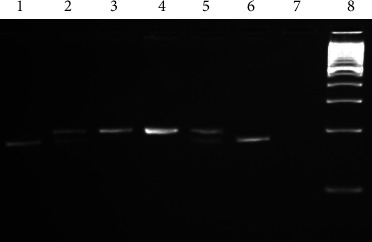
Genotypic profiles of the *C677T* polymorphism of *MTHFR* on agarose gel. Lane 8 represents DNA ladder; lanes 3 and 4 represent CC wild type; lanes 2 and 5 represent CT heterozygous; lanes 1 and 6 represent TT-mutated homozygous.
